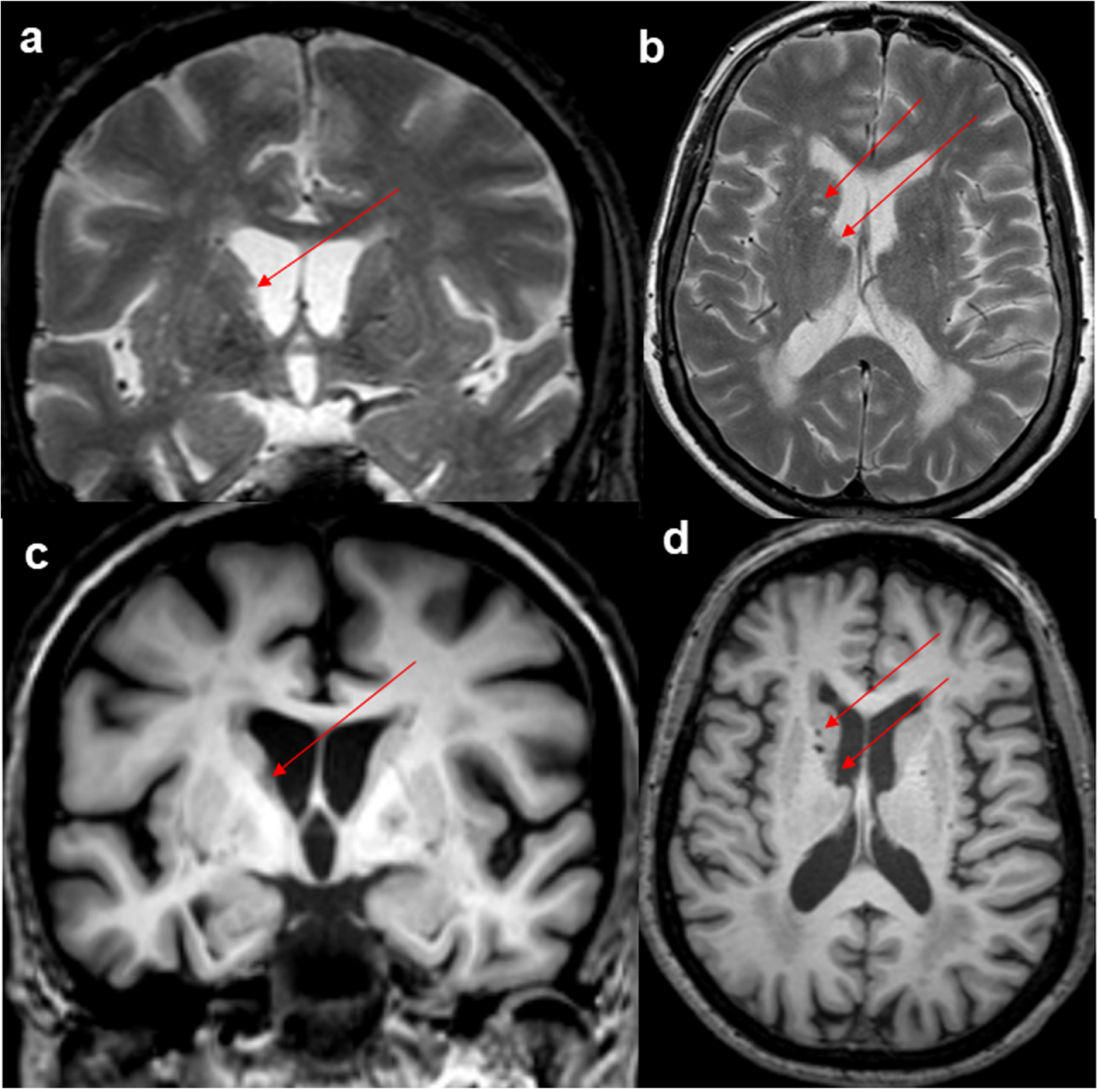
Brain MRI (3 T) of case 8. Lacunar lesions in superior-anterior-medial surface of right thalamus in the proximity inferior surface of caudal nucleus (arrows) (A: coronal T2* weighted image and B: axial T2 weighted image). Microvascular leukoencephalopathy (Fazekas grade 3). No hemosiderin accumulation in basal ganglia in T2* weighted images. No medial temporal lobe atrophy (C and D: coronal and axial T1 weighted images)
